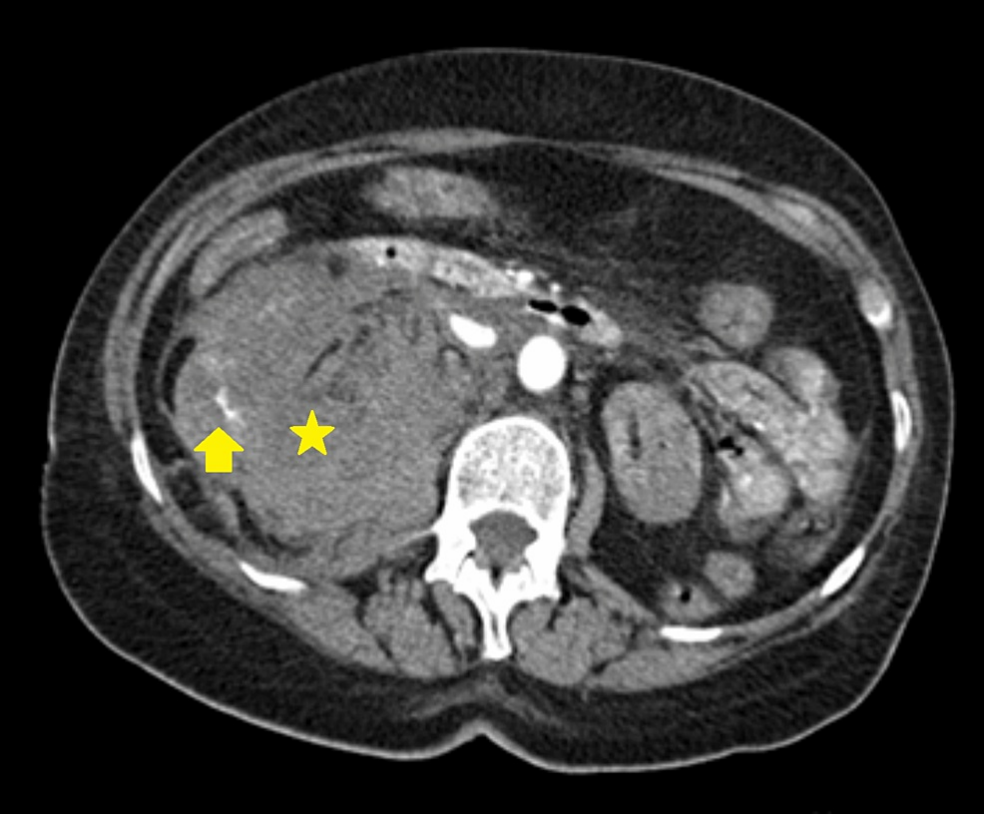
Right-sided renal and retroperitoneal hematoma - CT scan, transversal cut The hematoma (yellow star) occurred as a late complication of the kidney biopsy - four days after the procedure. The yellow arrow points toward the active extravasation of material, which suggests active hemorrhage.
